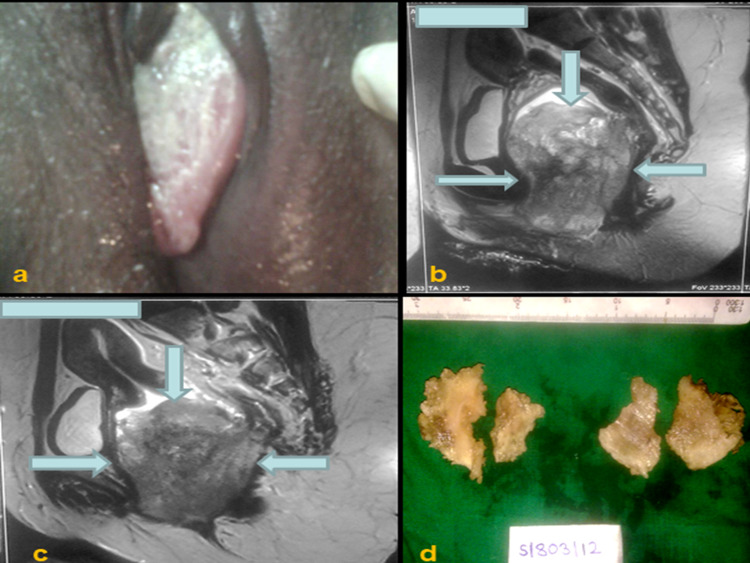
(a) Clinical image showing non-pigmented polypoid tumor arising from the lower third of the vagina and involving posterior and lateral wall. (b and c). MRI shows a large heterogenous hyperintense lesion at the vaginal and cervical region in T2-weighted images. (d) Gross showing non-pigmented grayish-white tissue biopsy pieces.
